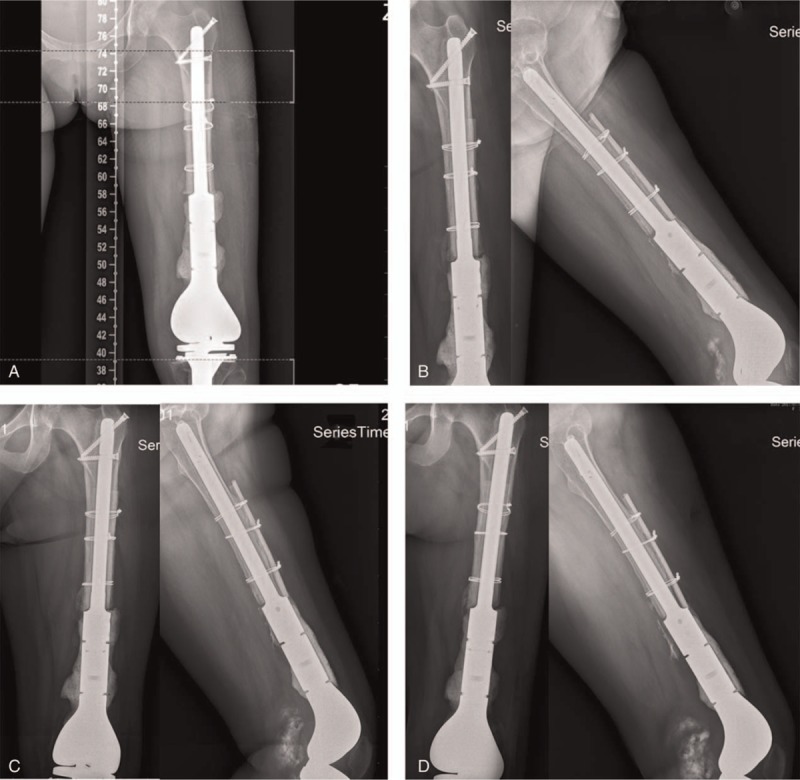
Postoperative x-ray images. A, Postoperative x-ray image. B, 6-Month follow-up. C, 16-Month follow-up. D, 22-Month follow-up.
